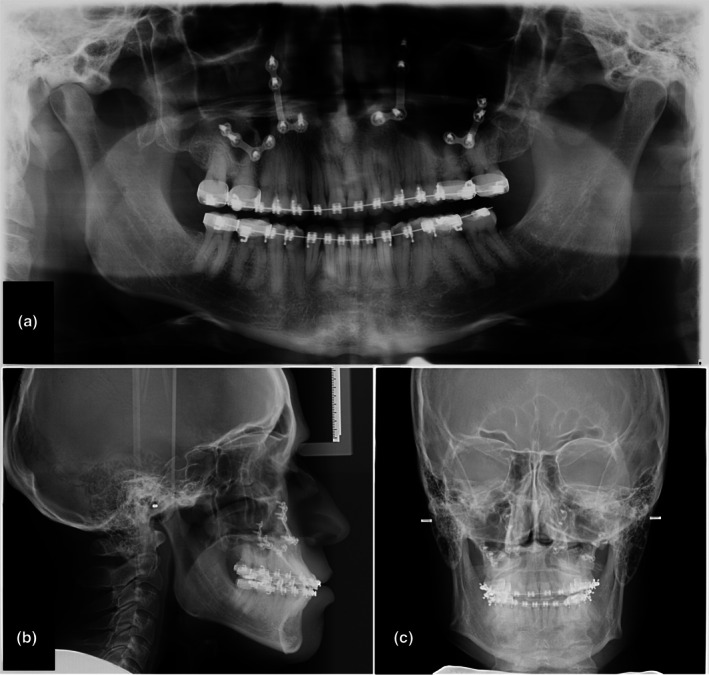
Postoperative digital radiographic assessments of the same patient following orthognathic surgery: (a) panoramic radiograph, (b) lateral cephalometric projection, and (c) posteroanterior (frontal) cephalometric projection.
